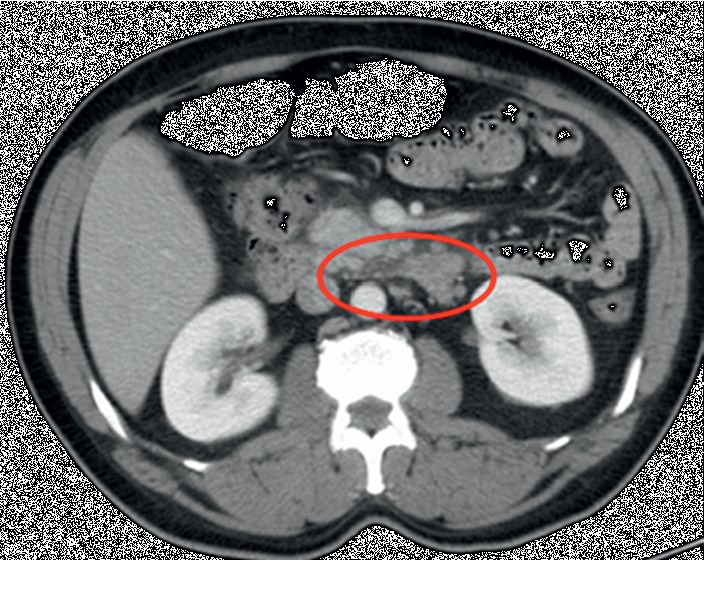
CT scan after initial presentation of acute pancreatitis.
